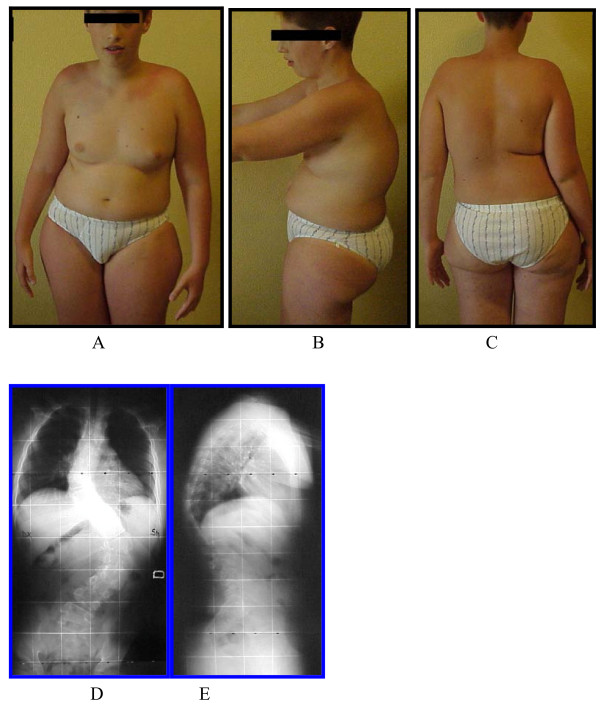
Preoperative clinical and radiographic picture. C.M., male, born in 1993, operated on in 2005; severe kyphoscoliosis in PWS.
